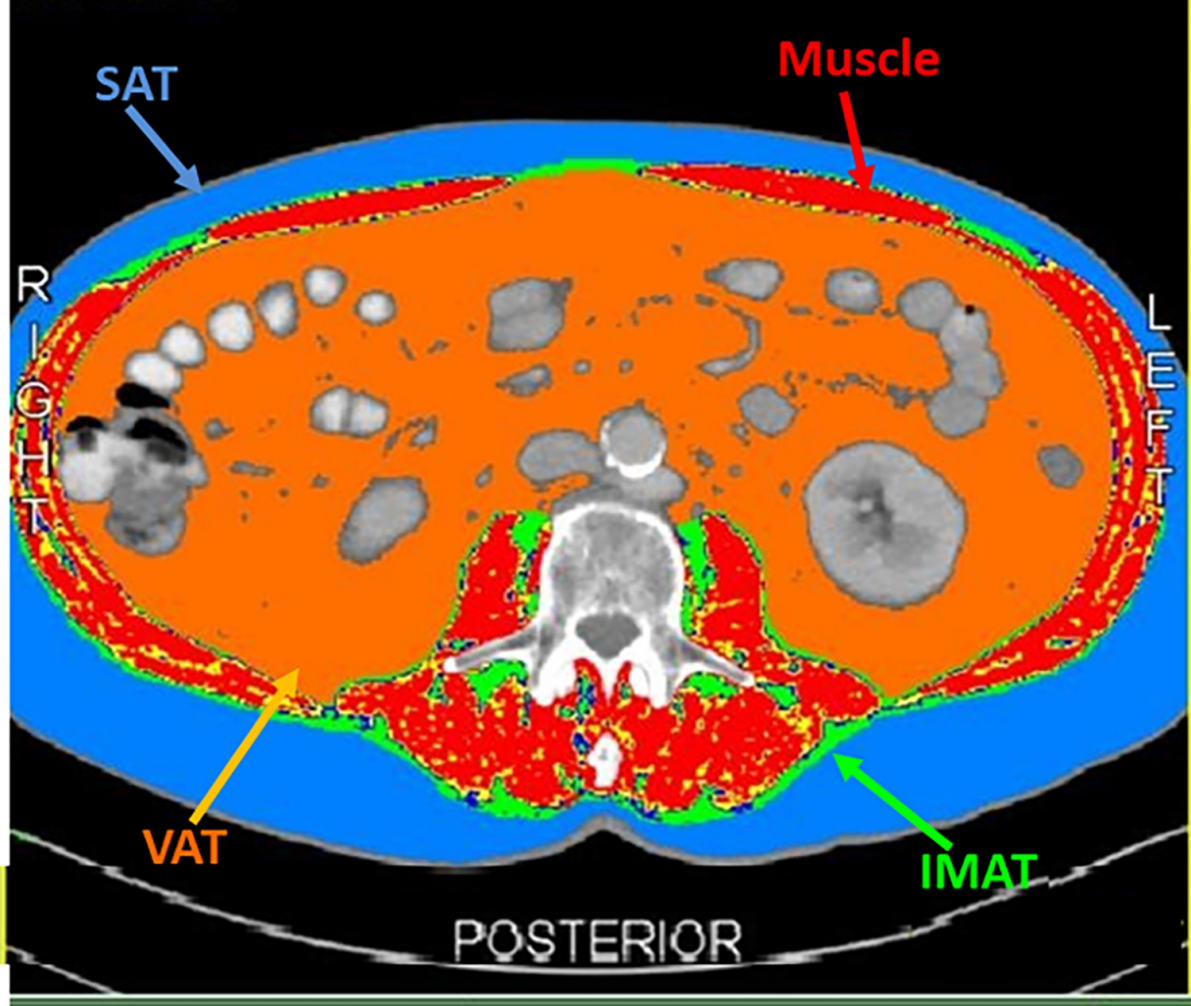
SliceOmatic tomovision analysis of the computed tomography scan of a male patient distinguishing the different context of Visceral Adipose Tissue (VAT), Intramuscular Adipose Tissue (IMAT), Subcutaneous Adipose Tissue (SAT) and skeletal muscle according to their differential Hounsfield Units (HU) references.
